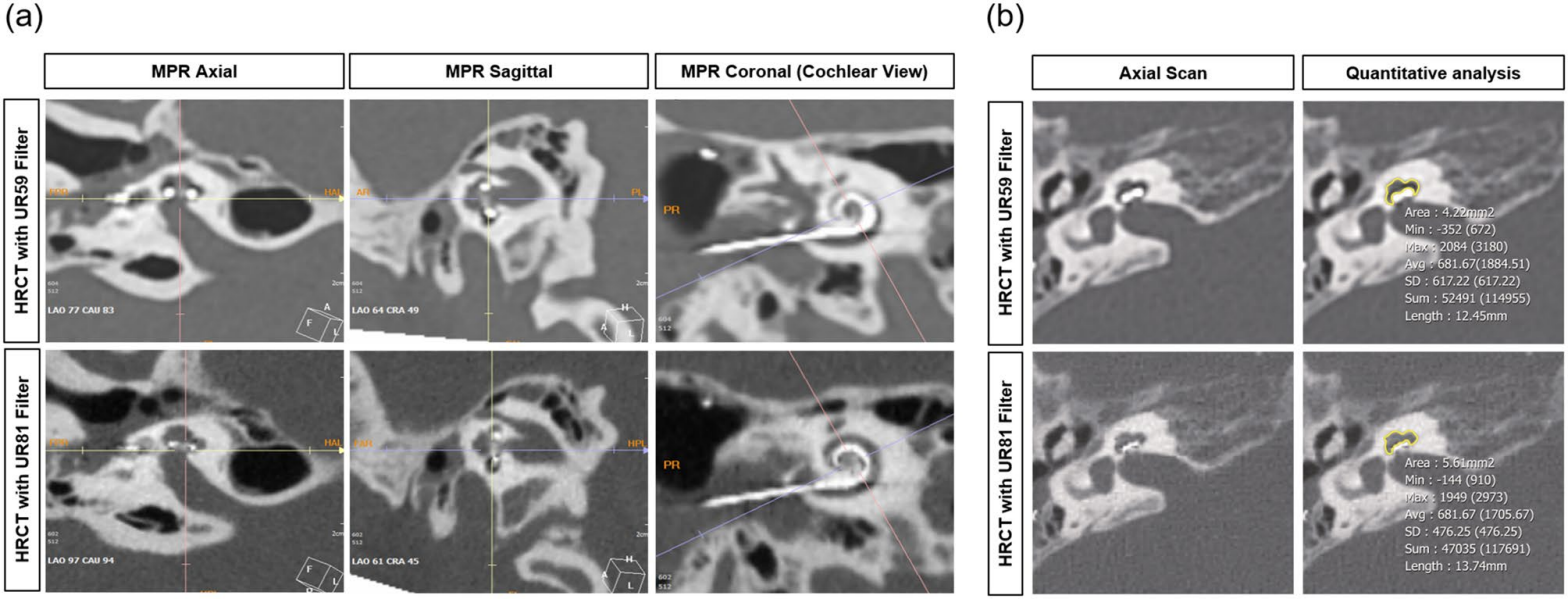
Reformatted conventional computed tomography along the plane of the “Cochlear View” by a multiplanar reconstruction algorithm. (a) Based on the “Cochlear View”, qualitative assessments of the slim modiolar electrode array were compared between the two resolution indices of UR59 (low resolution index, upper panel) and UR81 (high resolution index, low panel) in terms of imaging sharpness and overall subjective image quality. (b) For quantitative assessments, the noise level of the region of interest (yellow line) obtained by the middle turn of the cochlea was compared between conventional computed tomography with two different tomographic resolutions using ultra-high kernel specification. HRCT, high-resolution computed tomography; MPR, multiplanar reconstruction.
